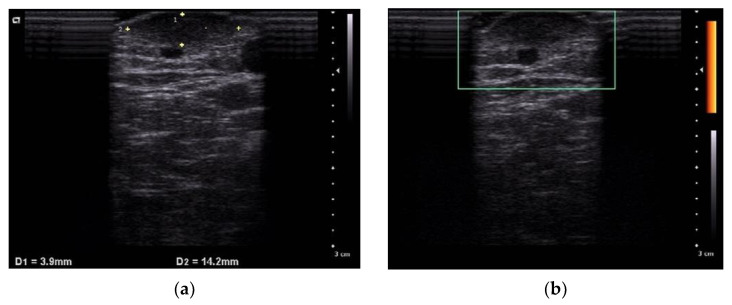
Echo ultrasonography shows a slightly hypoabsorbent nodule with indistinct borders (a). Doppler image; No internal blood flow is observed (b).
